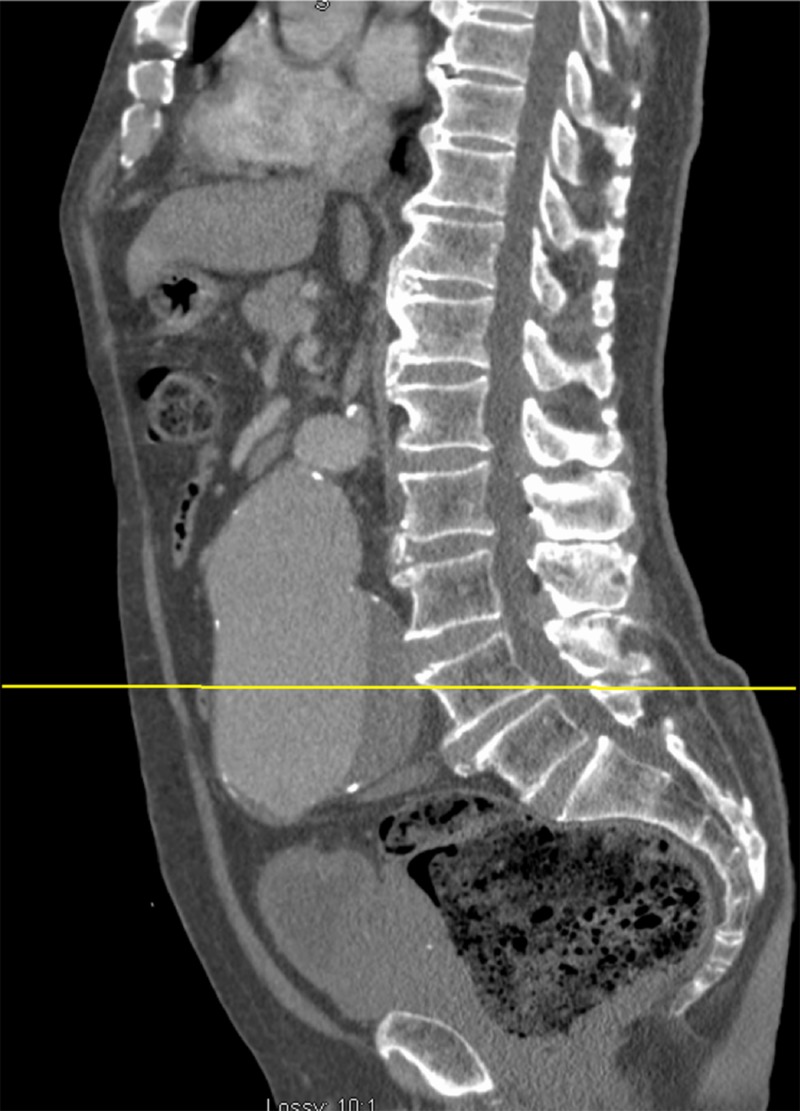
Contrast-enhanced midline sagittal CT image showing a 9.5 cm infrarenal AAA with mural thrombus The yellow horizontal line denotes the level of the axial image in Figure 1. AAA: abdominal aortic aneurysm; CT: computed tomography
